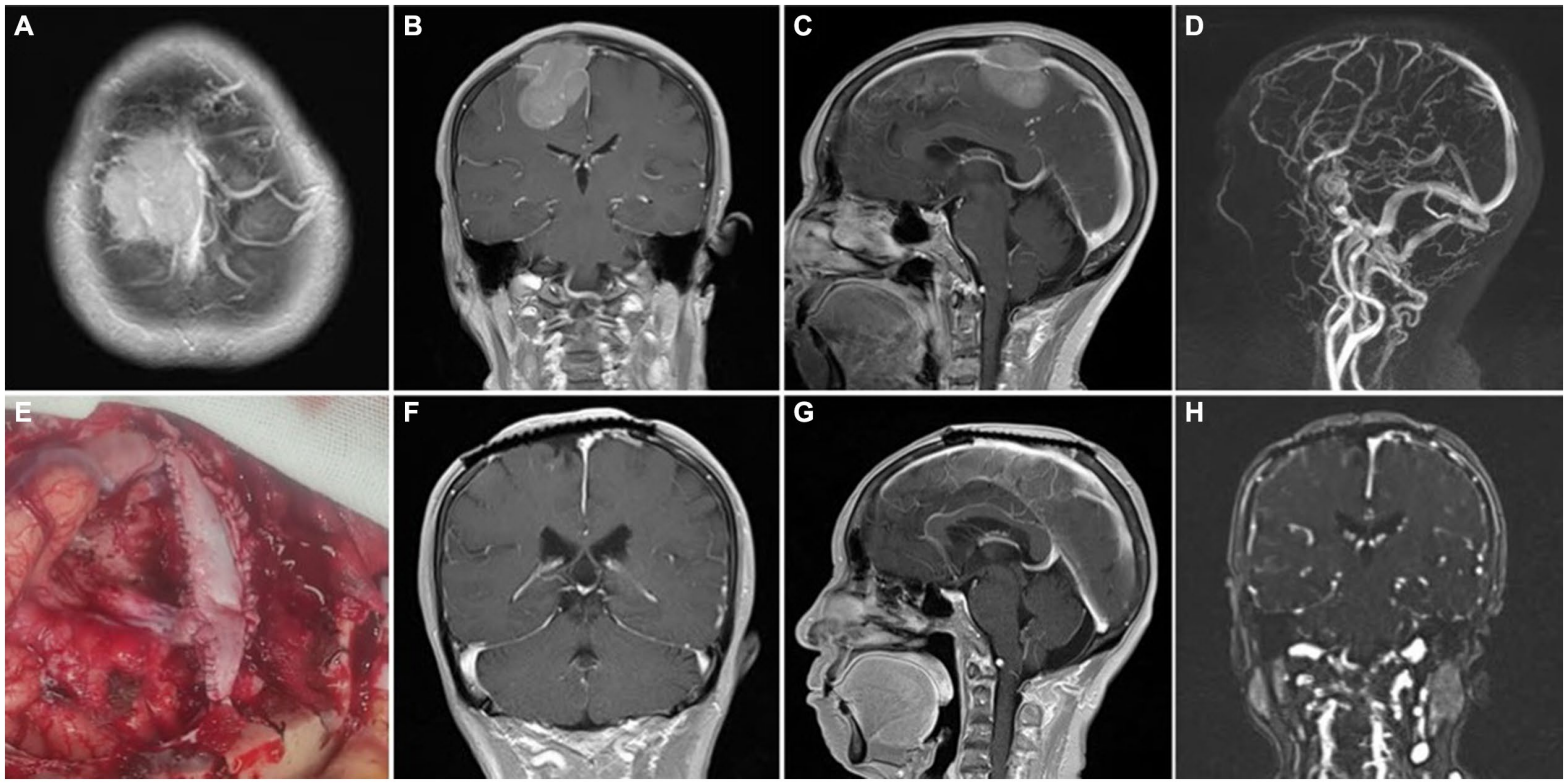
Representative case 3, type III (complete sinus obstruction). (A–C) Preoperative enhanced MRI images. (D) Preoperative MRV images. (E) Intraoperative patch reconstruction of the sinus wall. (F,G) Postoperative enhanced MRI images with concurrent titanium mesh repair of the cranial defect. (H) Postoperative MRV images.
